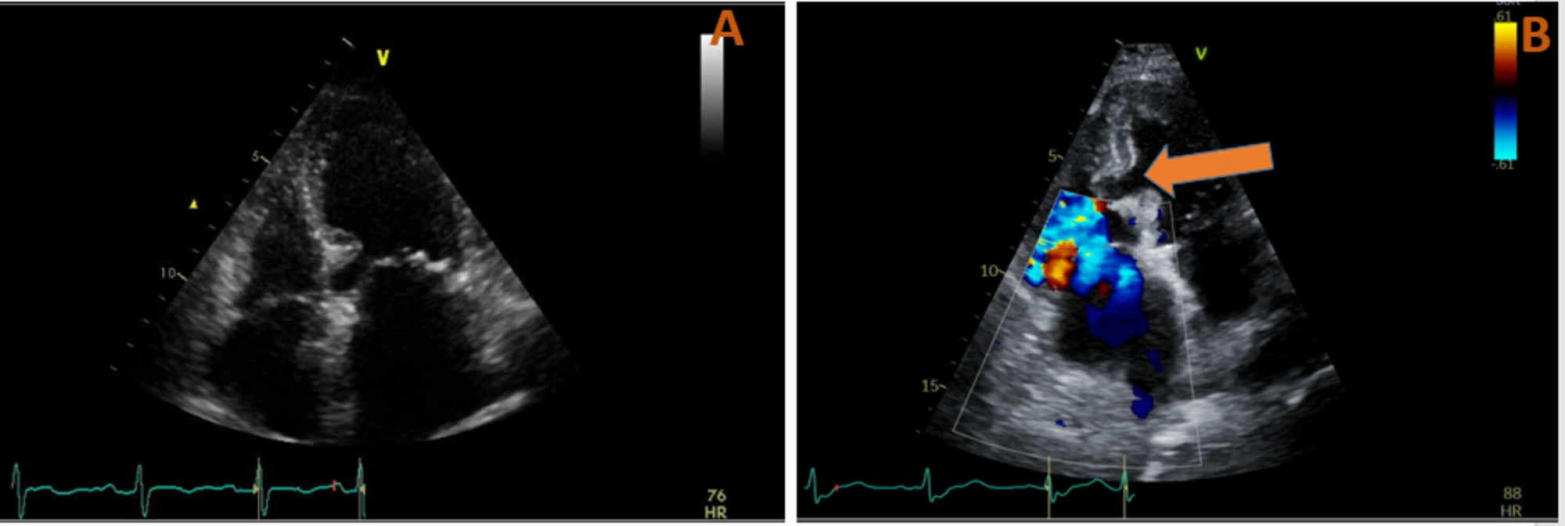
(A) Echocardiogram before cardiac catheterization showed reduced EF (35-40%) with an intact interventricular septum. (B) Doppler echocardiogram after a cardiac catheterization showed a significant reduction of EF (<20%) with new interventricular septum rupture, as shown by the white arrow. EF, ejection fraction
